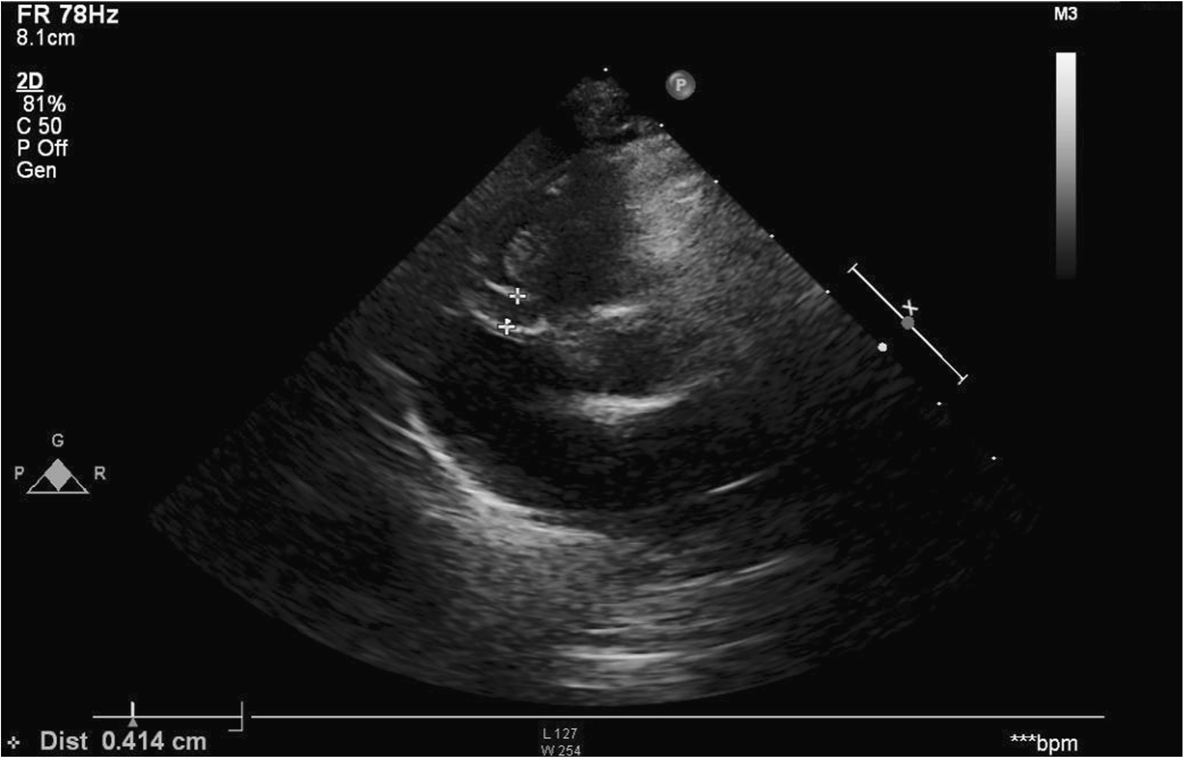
Echocardiography. After second treatment with intravenous immunoglobulin the patient developed ectasia of left coronary artery and a worsening of the dilatation of right coronary artery.
